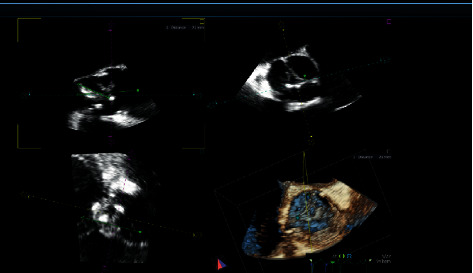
Echocardiogram images showing vegetations (densities) on the aortic valve with severe aortic regurgitation (arrow shows the vegetations).
